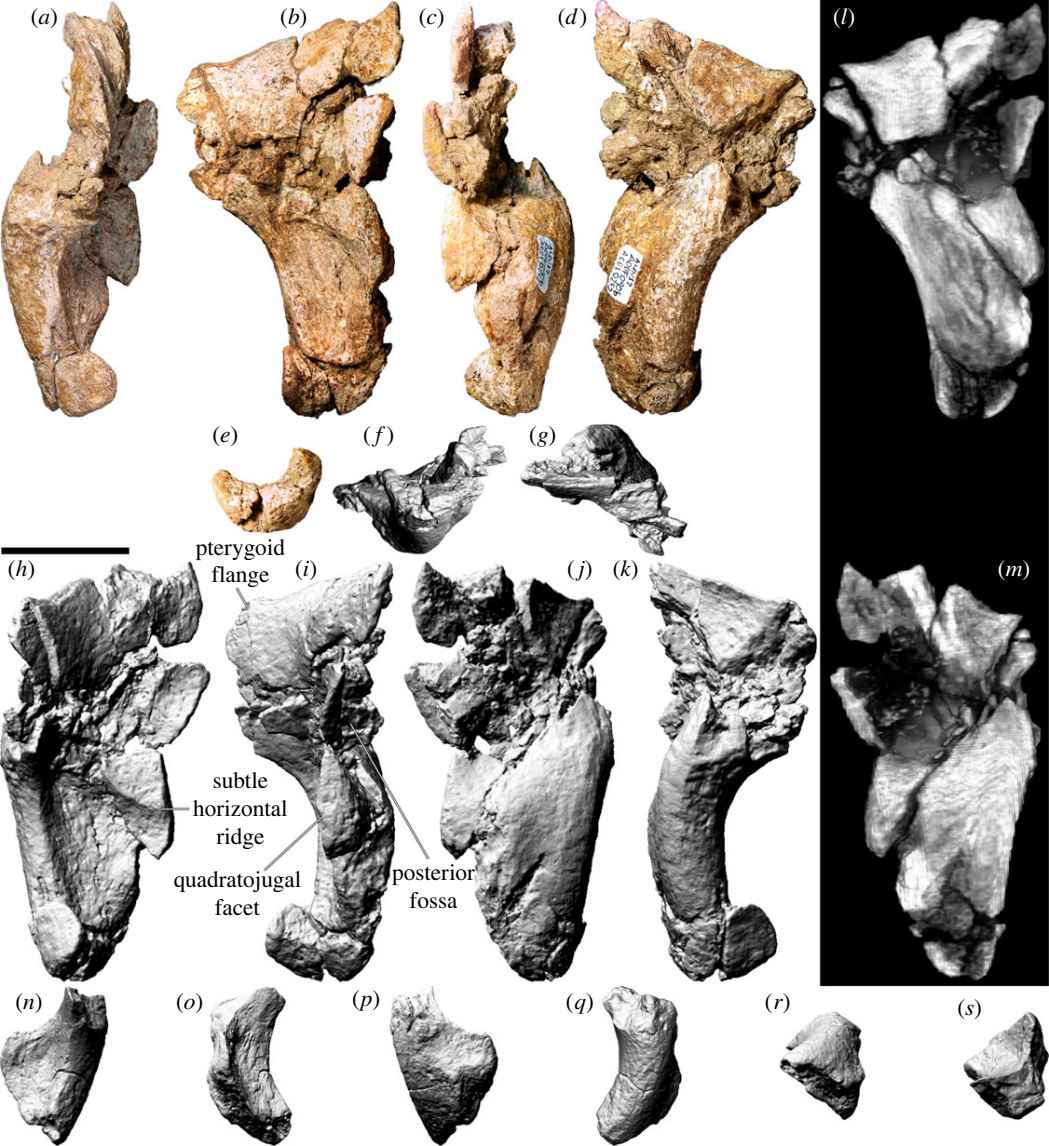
Diamantinasaurus matildae* referred quadrates (AODF 0906). (*a–e*) Photographs of the left quadrate in (*a*) anterior, (*b*) lateral, (*c*) posterior, (*d*) medial and (*e*) ventral views. (*f–k*) Three-dimensional models (derived from surface scans) of the left quadrate in (*f*) ventral, (*g*) dorsal, (*h*) anterior, (*i*) lateral, (*j*) posterior and (*k*) medial views. (*l,m*) Three-dimensional models derived from CT scans of the left quadrate in (*l*) lateral and (*m*) medial views. (*n–s*) Three-dimensional models (derived from surface scans) of the right quadrate in (*n*) anterior, (*o*) lateral, (*p*) posterior, (*q*) medial, (*r*) dorsal and (*s*) ventral views. Scale bar = 50 mm.
